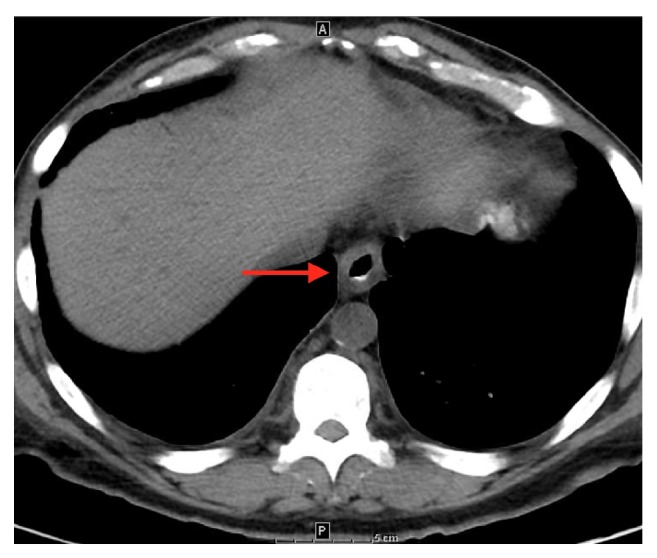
CT scan showing circumferential thickening of the distal esophagus.
